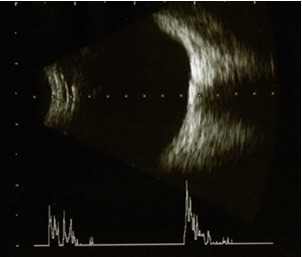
Ultrasonography left eye
